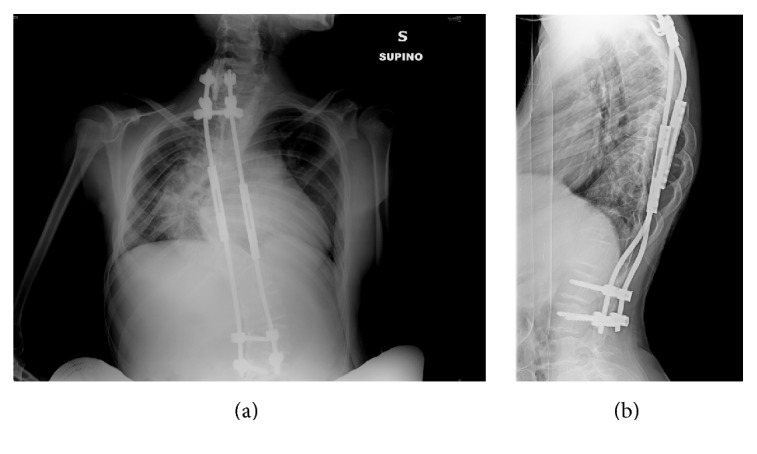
Anteroposterior (a) and lateral (b) chest X-ray showing patient's pronounced spinal curvature and dorsal hump. A provisional extensible distractor is in place.
